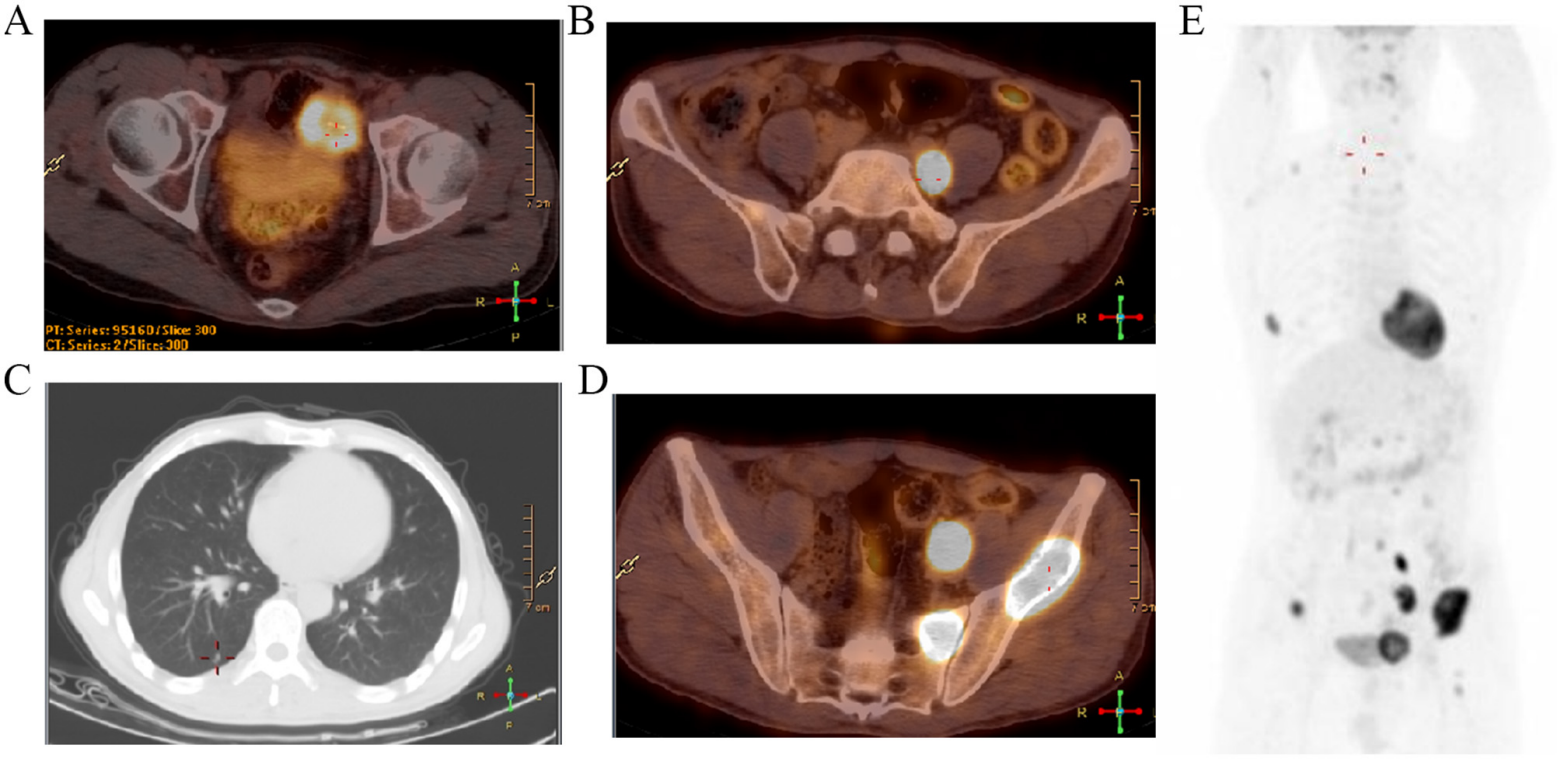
The results of CT/PET-CT. (A–C) High uptake localization of the left pelvis, retroperitoneal lymph nodes, and multiple micronodules in the lungs. (D) Osteolytic bone destruction and enhanced uptake in the pelvis and other sites. (E) Whole body PET-CT imaging.
